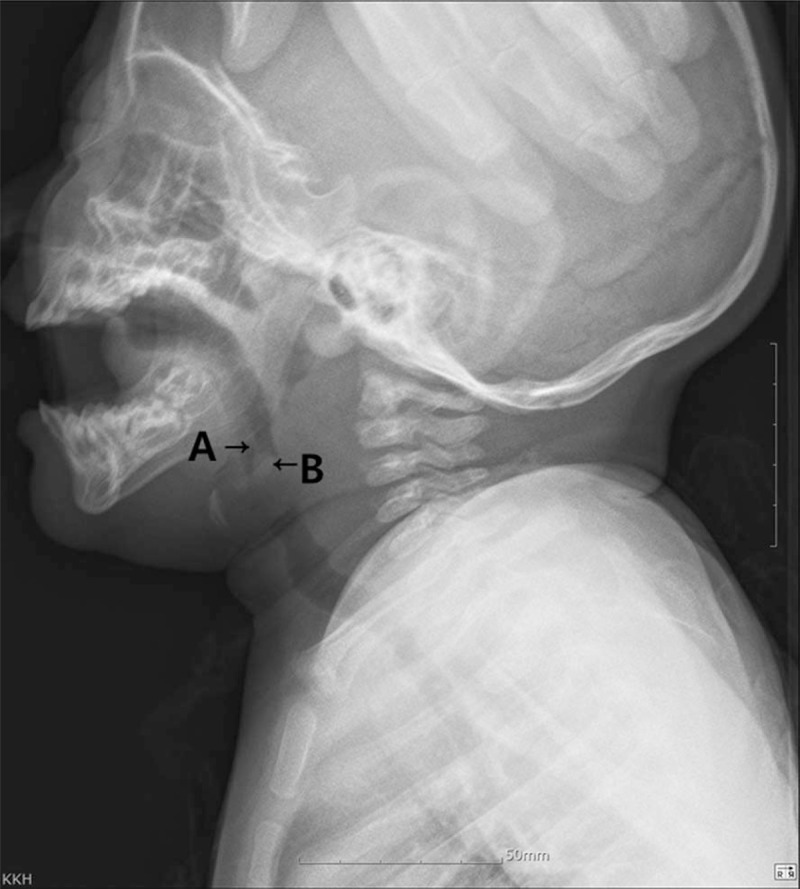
A neck soft tissue X-ray reveals the upper airway to be obstructed by the uvular cyst. A: uvula. B: epidermoid cyst.
